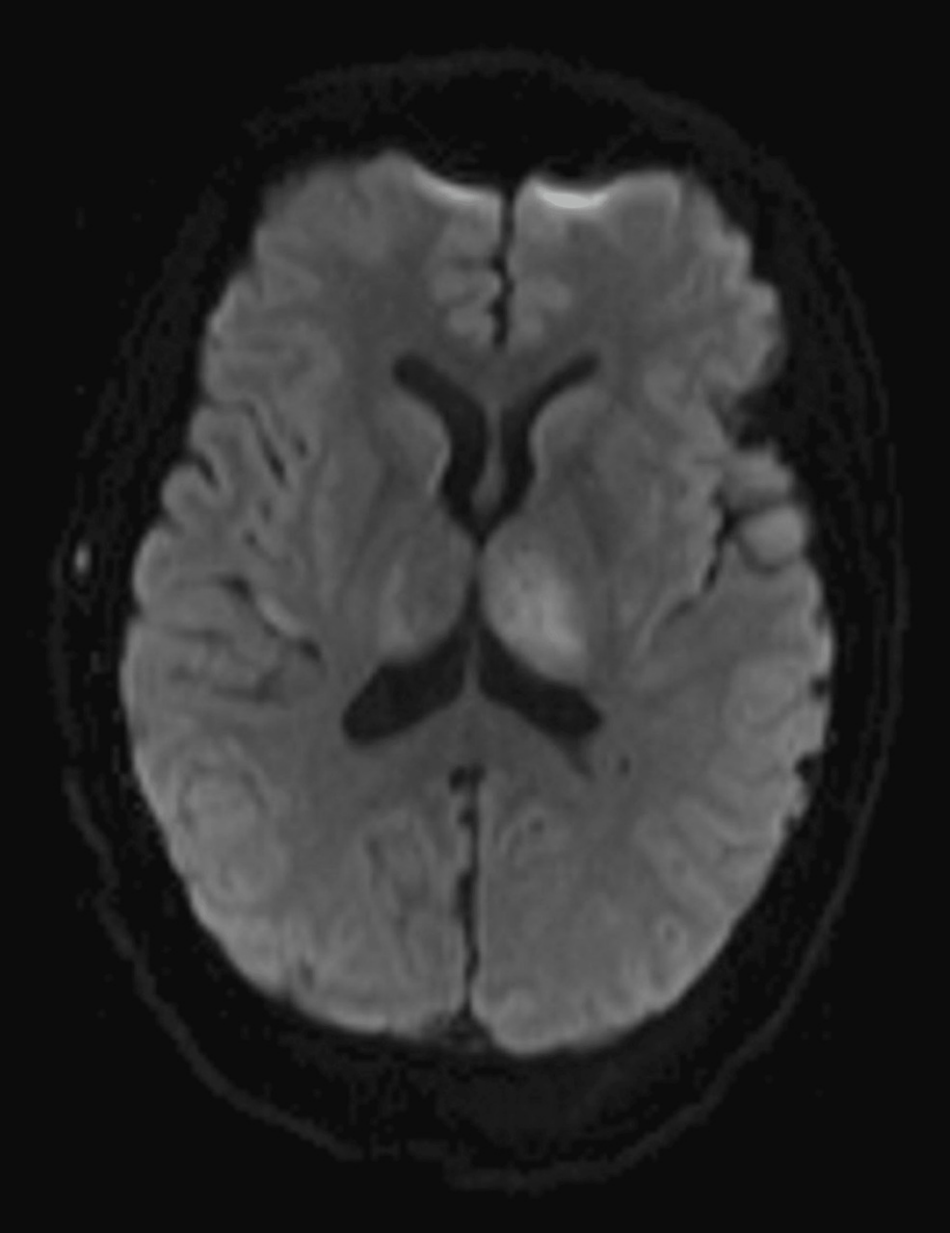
MRI of the brain DWI sequence in transverse view showing increased signal in bilateral thalamic structures, with the left thalamus being more severely affected indicating congestion. DWI: diffusion-weighted imaging.
